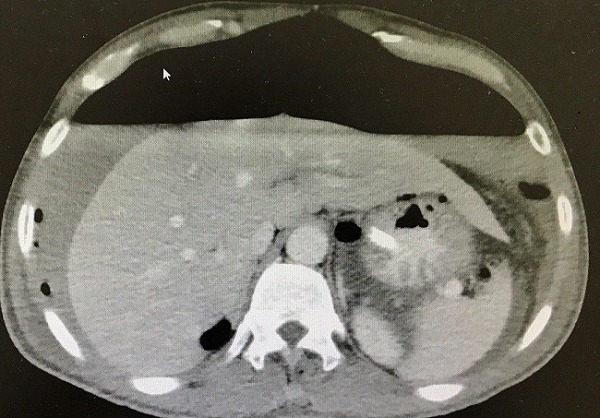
CT Scan was 2 days after omental Graham patch operation, showing massive hydro pneumoperitoneum
